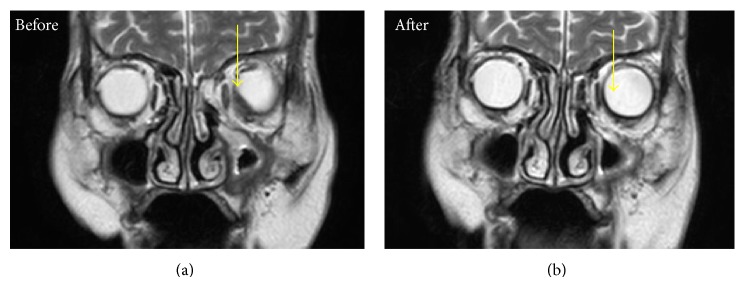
Coronal T2-weighted images showing a left choroidal tumor and space-occupying lesions in left paranasal sinus (arrow) before and 3 weeks after steroid treatment.
